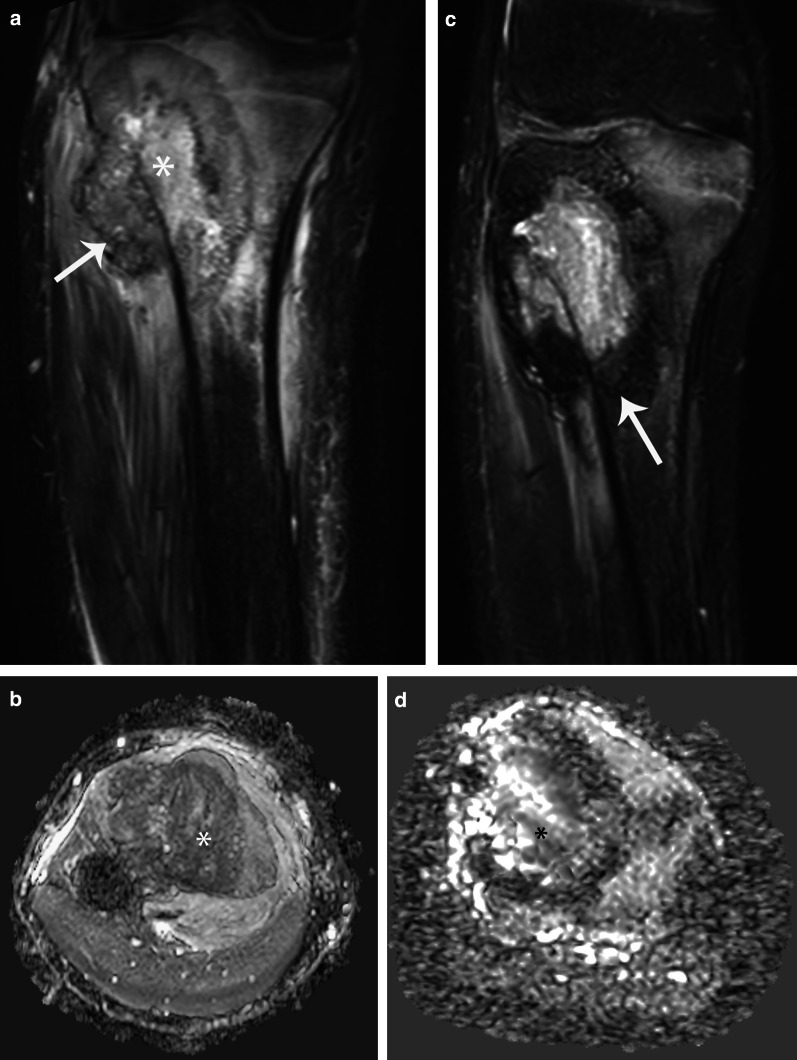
Osteosarcoma involving the proximal metaphysis of the tibia in a 13-year-old boy. a Coronal fat-suppressed T2-weighted MR image shows a heterogeneous bone lesion (*) associated with a soft-tissue mass (arrow). b Axial ADC MR image shows diffusion restriction within the tumor (*). c, d Post-chemotherapy MRI. c Coronal fat-suppressed T2-weighted MR image shows a peripheral hypointense rim in the tumor suggestive of calcification (arrow). d Axial ADC MR image shows decrease of the areas of diffusion restriction (*) compared to the initial study, indicating good treatment response
